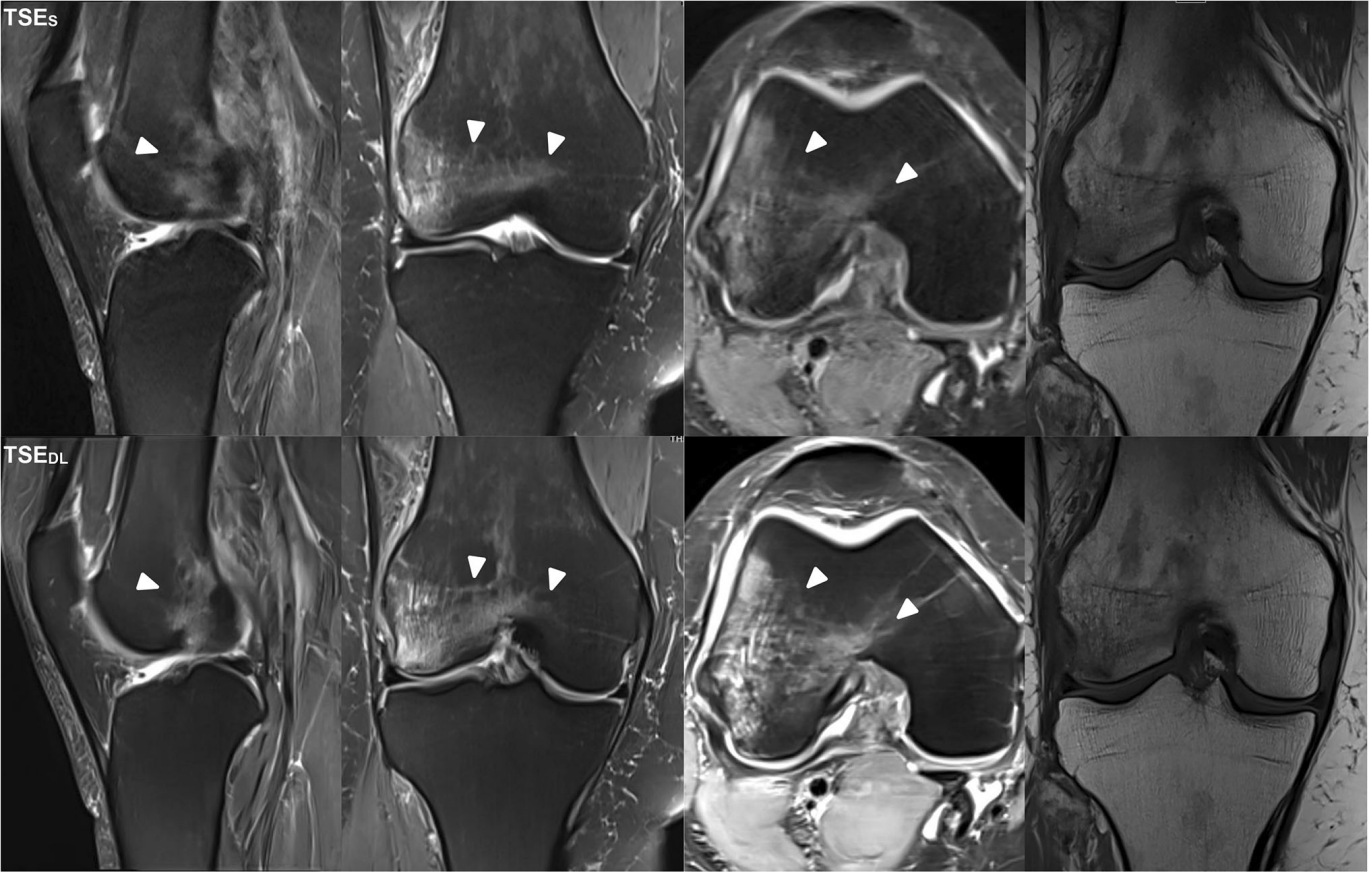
Image example of a standard and deep-learning-reconstructed PD TSE imaging of the knee at 3 T. This is an example of a comprehensive knee MRI at 3 T of a 46-year-old patient with pain in the medial side of the right knee after trauma. PD– and T1–weighted TSE_S_ (upper row) and TSE_DL_ (lower row) in different orientations are compared. TSE_DL_ provides higher image quality with lower extents of noise and improved sharpness of the anatomic structures. Note that bone marrow edema (white arrowheads) of the femoral condyle is clearly definable in both TSE_S_ and TSE_DL_
